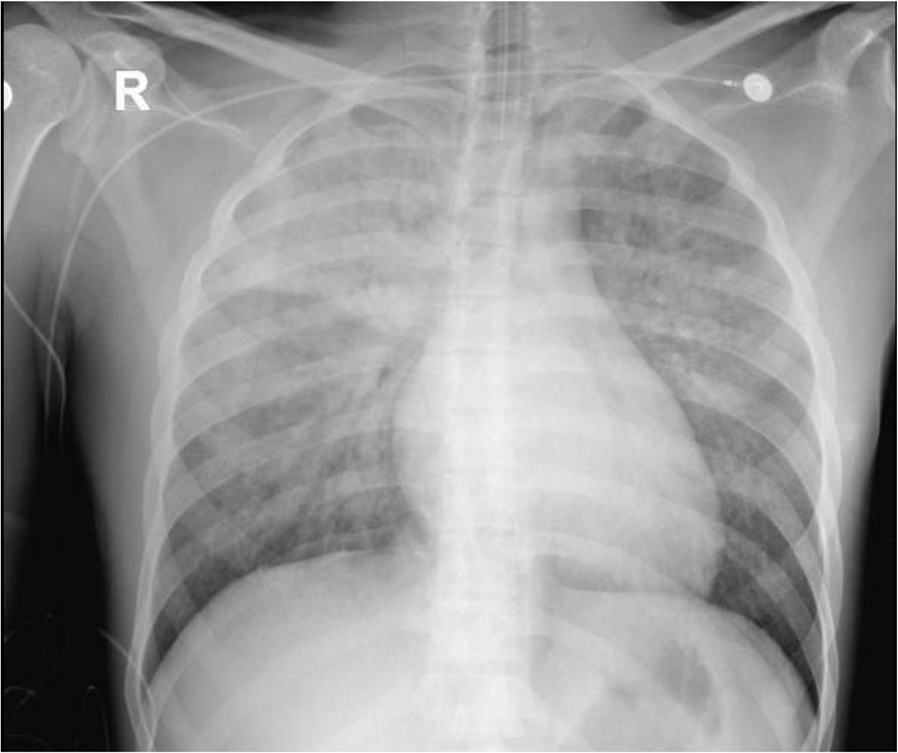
Chest X-ray at admission indicate bilateral pulmonary edema and normal heart silhouette
